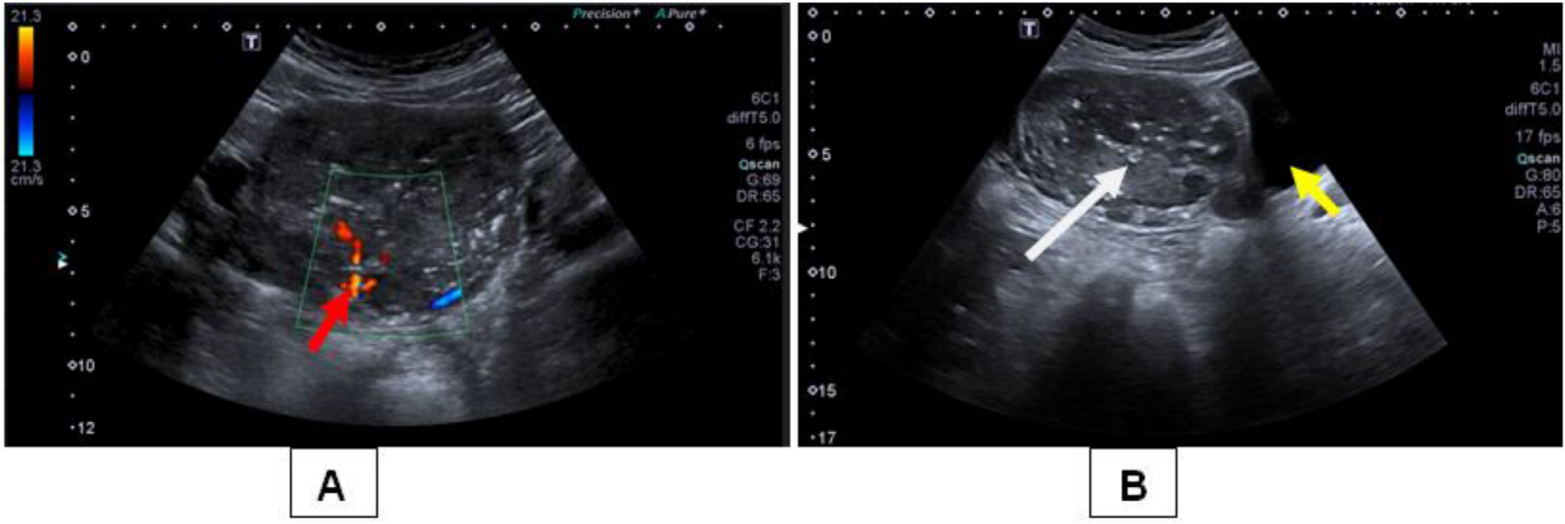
An ultrasound of the abdomen and pelvis revealed a 15-cm hypoechoic heterogeneous bean-shaped tumor (white arrow) above the bladder (yellow arrow) with internal vascularity (red arrow) and calcification.
